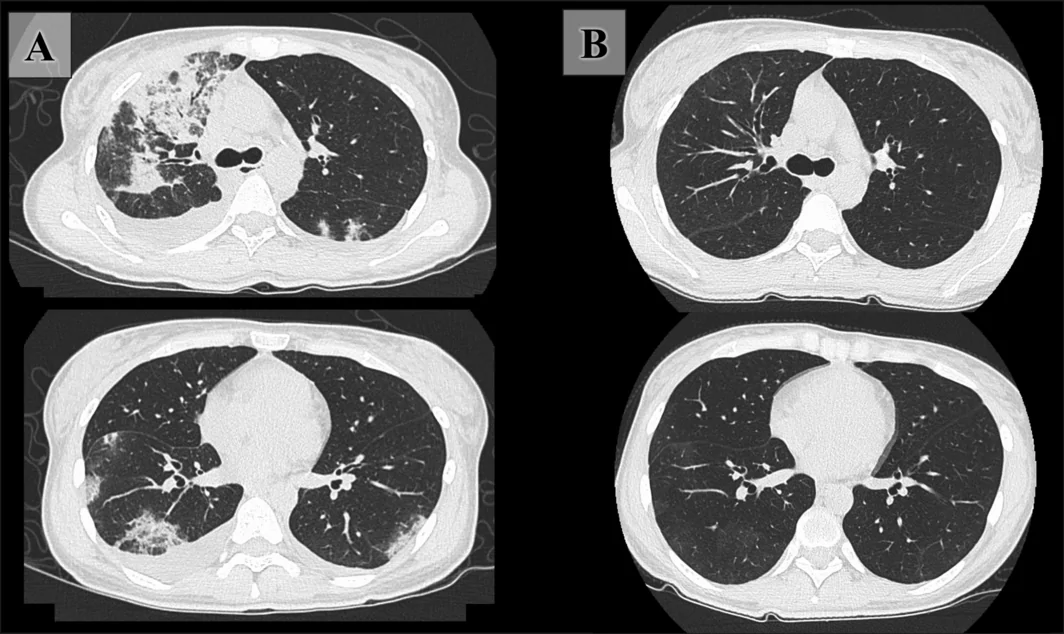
Chest CT images. (A) Chest CT image obtained at the first visit to our hospital showing diffuse consolidation, which was unilaterally predominant in the right upper lobe with pleural effusion. (B) A chest CT image obtained 42 days after stopping mesalazine showing no evidence of pneumonia.
